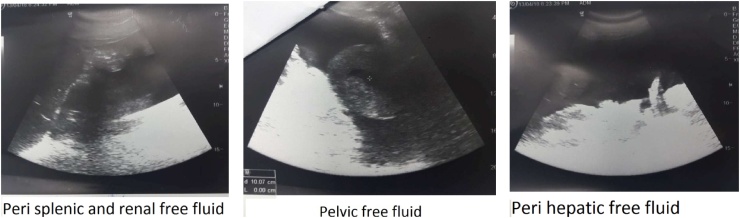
Abdominal and pelvic ultrasound showing marked ascites.
